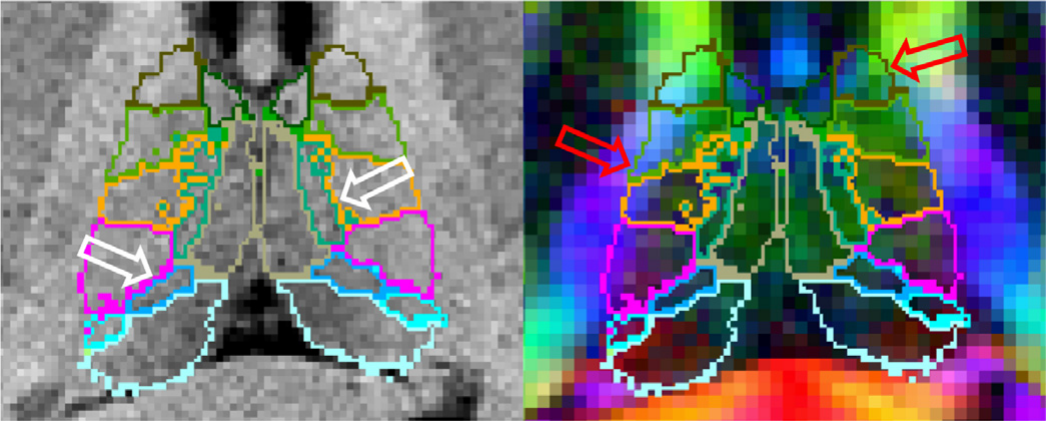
Thalamic segmentation of a T1-weighted structural MRI overlaid on the co-registered T1-weighted image (left) and a co-registered directionally encoded colour FA image (right). High contrast between medial and lateral thalamic regions on structural imaging improves the accuracy of these boundaries (white arrows). However, low contrast between the lateral thalamus and white matter causes over-segmentation into the internal capsule, which can easily be discerned in the colour FA image (red arrows). (For interpretation of the references to colour in this figure legend, the reader is referred to the web version of this article.)
